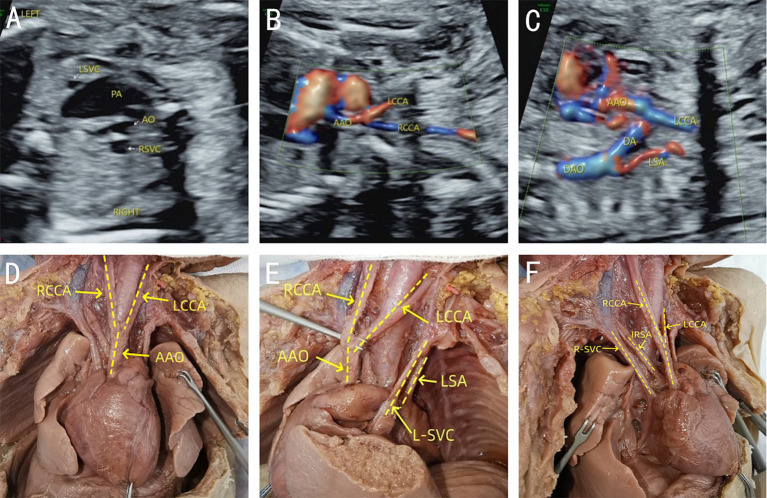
IAA Type B prenatal ultrasound and postpartum local pathological anatomy. (A) The pulmonary artery is significantly enlarged, and the diameter of the aorta is smaller than that of the pulmonary artery. (B) The ascending aorta is discontinuous with the descending aorta after it has given rise to two branches. (C) The left subclavian artery is connected to the descending aorta. (D–F) Following induced abortion, local pathological findings revealed malformation of the ascending aorta. After the ascending aorta gives rise to the right common carotid artery and the left common carotid artery, it loses continuity with the descending aorta. The right subclavian artery connects to the pulmonary artery, and double superior vena cavae are visible.
